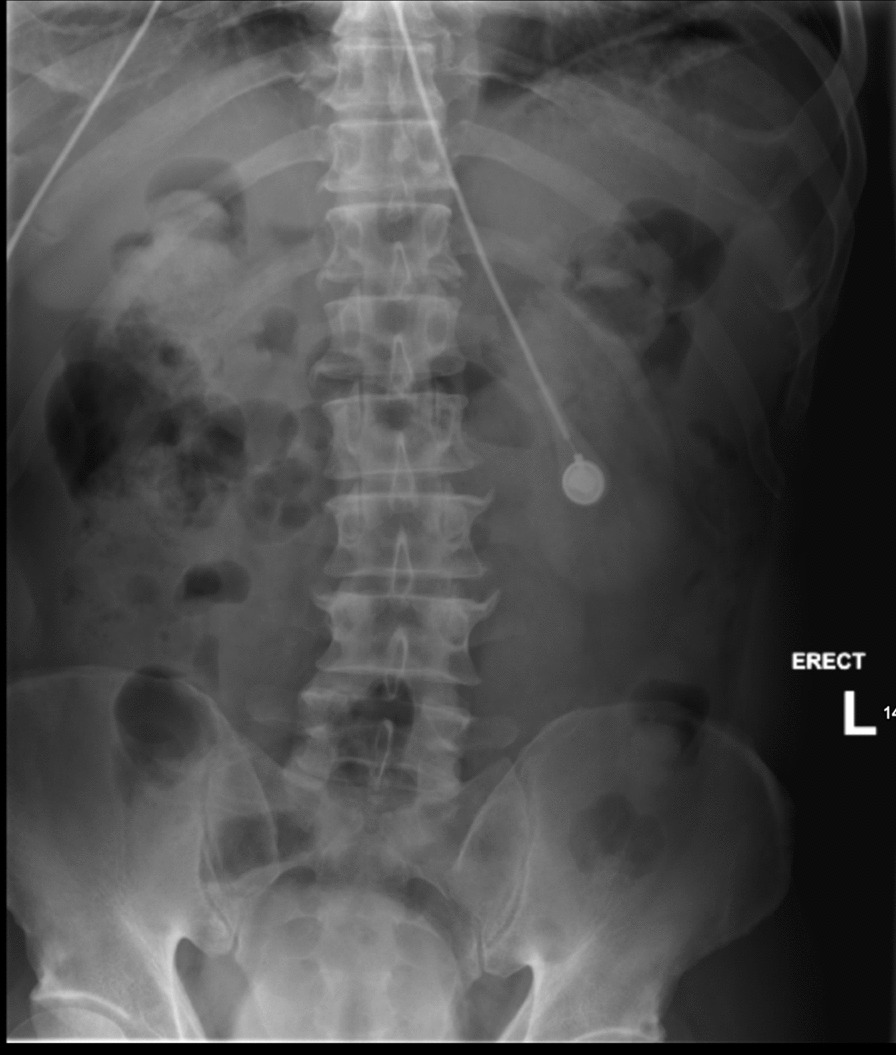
Anteroposterior X-ray of lumbar spine demonstrating no fractures or dislocations
